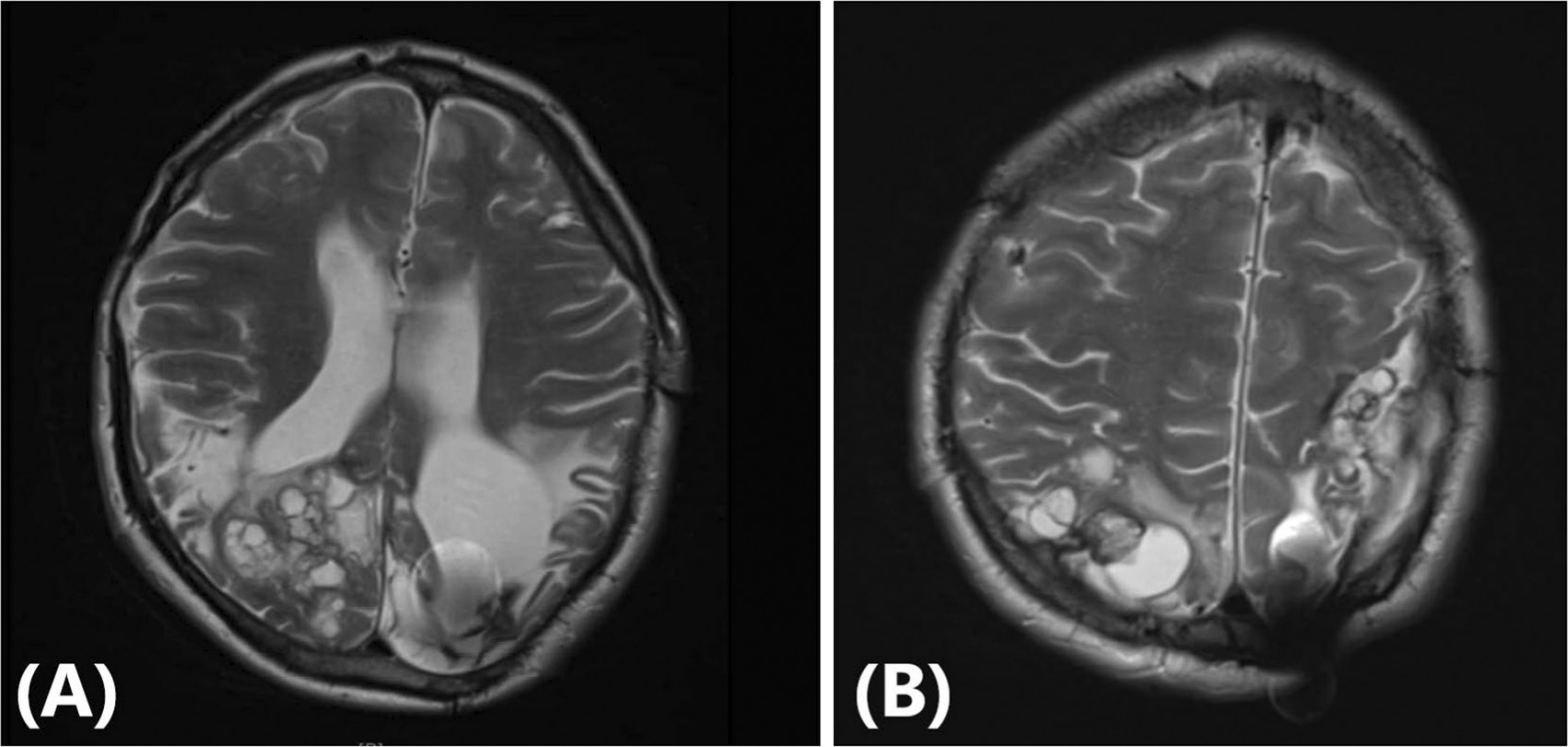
Axial T2‐weighted Brain MRI of a 35‐year‐old Male with Multi‐Organ Hydatid Cyst. (A) There are a few clusters of varying size cysts with high internal signal and thin hypo‐signal wall and without solid components associated with surrounding vasogenic edema at white matter of the right occipital lobe with pressure on the occipital horn of the right lateral ventricle (cut level: occipital horns of the lateral ventricles). (B) Multiple hydatid cysts are visible in the right occipital lobe, characterized by hyperintense signals with thin hypointense walls. The largest cyst exerts a mass effect on the occipital horn of the right lateral ventricle. Significant surrounding vasogenic edema is also noted (cut level: posterior periventricular white matter and occipital lobe). MRI, magnetic resonance imaging.
